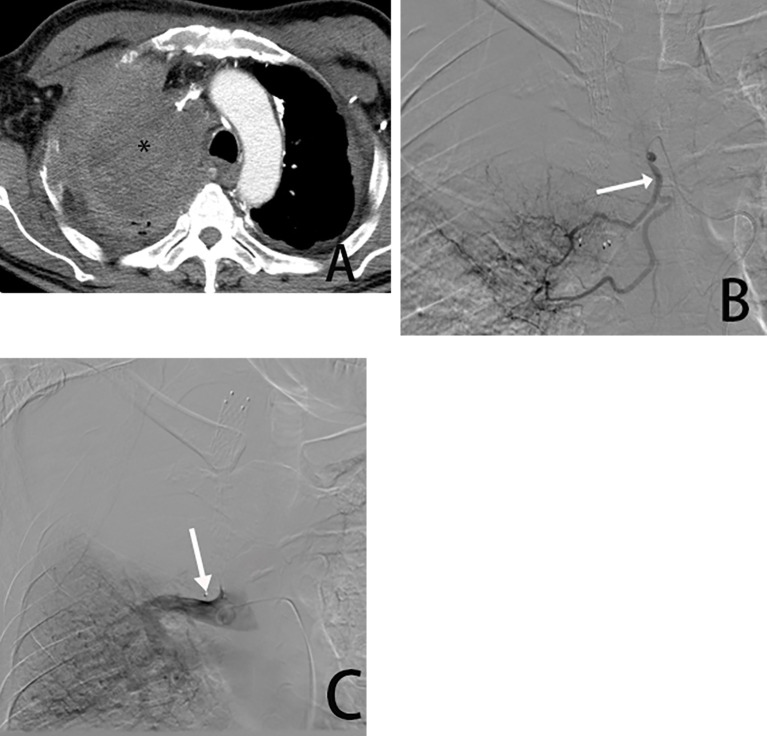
Male, 54 years old, tumor was found in the right upper lung. (A) is a CT cross-sectional view showing the right upper lung tumor (*). (B) is a right bronchial arteriogram (→) showing that the right lung tumor is supplied by the bronchial artery. (C) is a pulmonary arteriogram showing that the pulmonary artery was not involved in the blood supply(→) and was occluded due to compression by the tumor encompassing it.
